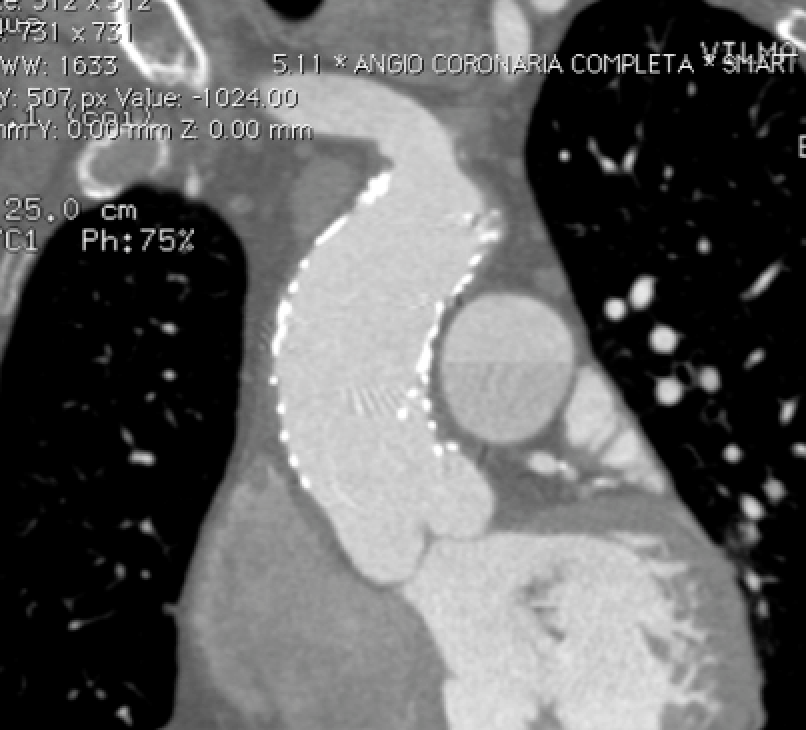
Computed tomography post stent graft in ascending aorta.
